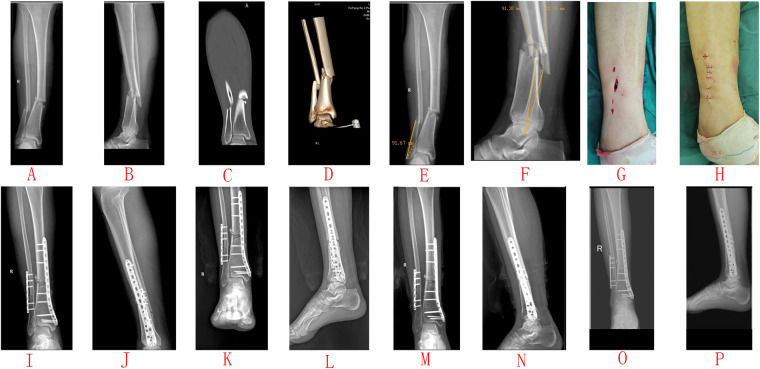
A 51-year-old male with a right tibiofibular fracture (weber type C), treated using the STMIPO technique for the fibula fracture. (A,B) Preoperative anteroposterior and lateral x-rays; (C) (D) 3D CT scan of the tibia and fibula; (E,F) Preoperative measurement of relevant indicators; (G,H) Surgical incision; (I,J) Postoperative anteroposterior and lateral x-rays; (K,L) Anteroposterior and lateral x-rays one month postoperative; (M,N) Anteroposterior and lateral x-rays three months postoperative; (O,P) Anteroposterior and lateral x-rays six months postoperative.
